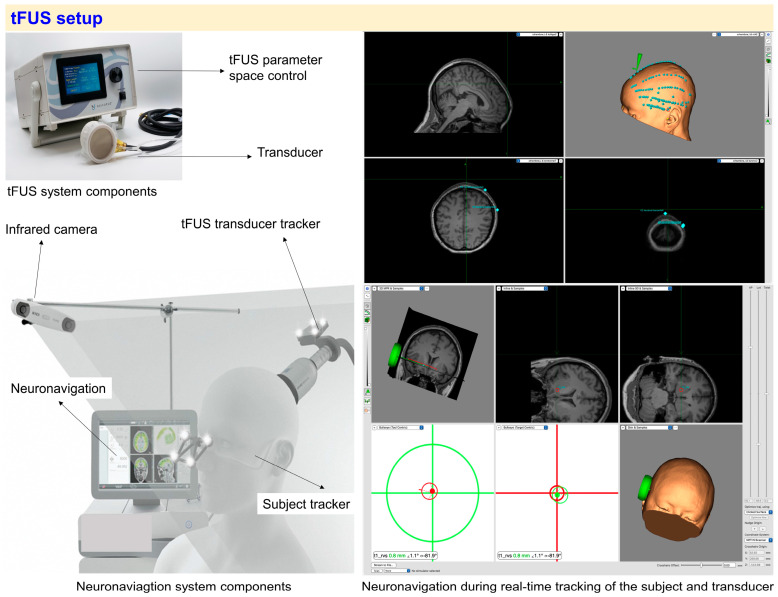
Overview of key components of the tFUS setup. (Left panel): The top left panel displays the main components of the tFUS setup, including the tFUS parameter space control unit and its transducer (image adapted from the BrainBox manufacturer, Cardiff, UK). The lower left panel shows the neuronavigation system, featuring infrared cameras for subject tracking and transducer positioning to assist in targeting during stimulation (image adapted from the NEUROLITH-TPS manufacturer, Tägerwilen, Switzerland). (Right panel): An example of real-time neuronavigation while tracking the subject and transducer with an infrared camera (images created using Brainsight software V2.5.3, Montréal, Canada). The top right panel shows scalp points (in green) that are taken for coregistering the brain MRI with the subject’s head position in real time. The bottom right panel displays the target and transducer positions, which are utilized to ensure an accurate and optimized stimulation.
